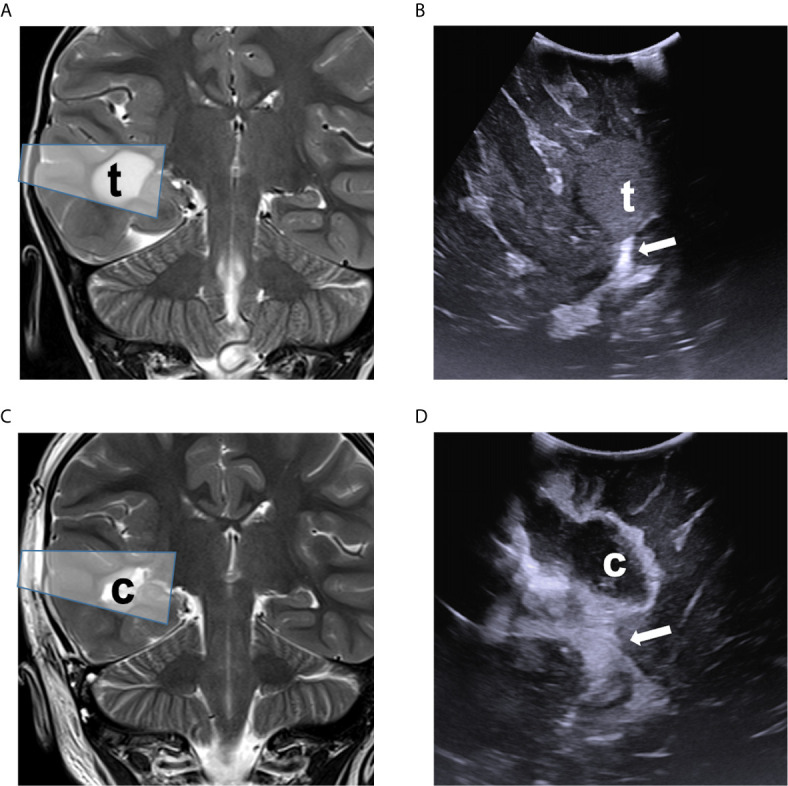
Comparison of ioUS with pre- and post-operative MRI images of a right temporal low-grade glioma. Pre-operative (A) and post-operative (C) coronal T2-weighted MRI sequence demonstrating the lesion (t) and surgical cavity (c) with ioUS approximate field of view (shaded box). Intraoperative US view of the same is shown before dural opening (B) and after resection (D), documenting the spatial relationship with the choroid plexus (white arrow).
